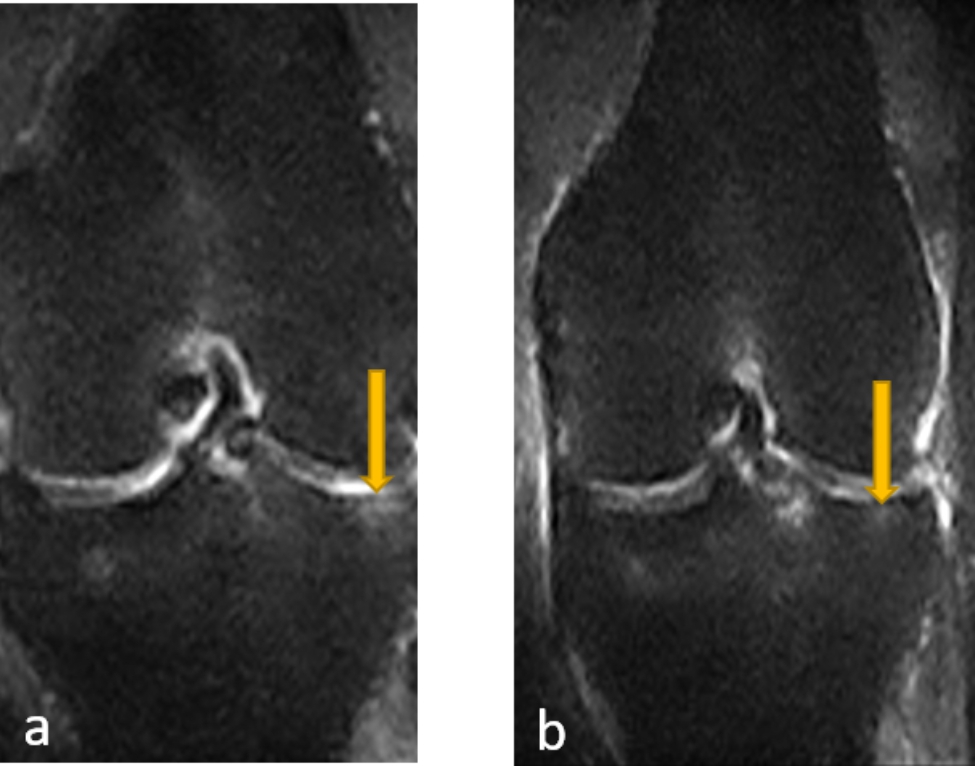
Representative MRI outcomes before injection (a) and 12 weeks after injection (b), indicating subchondral bone edema improvement
